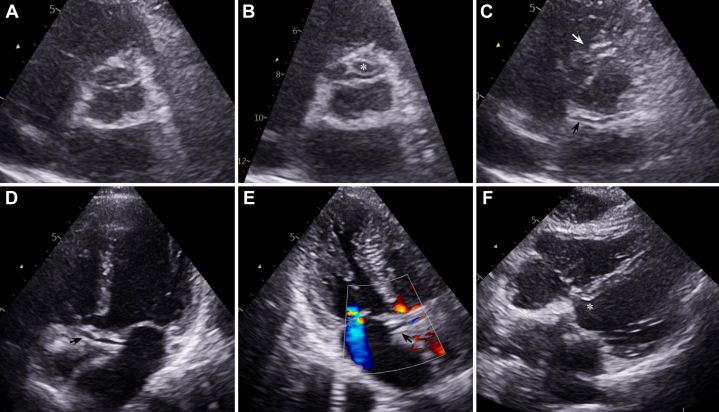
Origin and Course of Anomalous Coronary Arteries on TTE (A) Parasternal short-axis (PSAX) view showing a tricuspid aortic valve with normal characteristics. (B) Slightly higher PSAX view showing an intimal dissection flap in the RCS (asterisk) and the LAD origin. (C) Supravalvular PSAX view showing the posterior course of the LAD (white arrow) and the retroaortic course of the LCx (black arrow). (D) Modified apical 5-chamber view demonstrating the “RAC sign” (retroaortic anomalous coronary), with the LCx (black arrow). (E) Apical 3-chamber view with color Doppler showing the retroaortic LCx with minimal internal flow (black arrow). (F) Subcostal 4-chamber view showing the intramyocardial anteroseptal course and angulation at the onset of the mid-LAD (asterisk).
